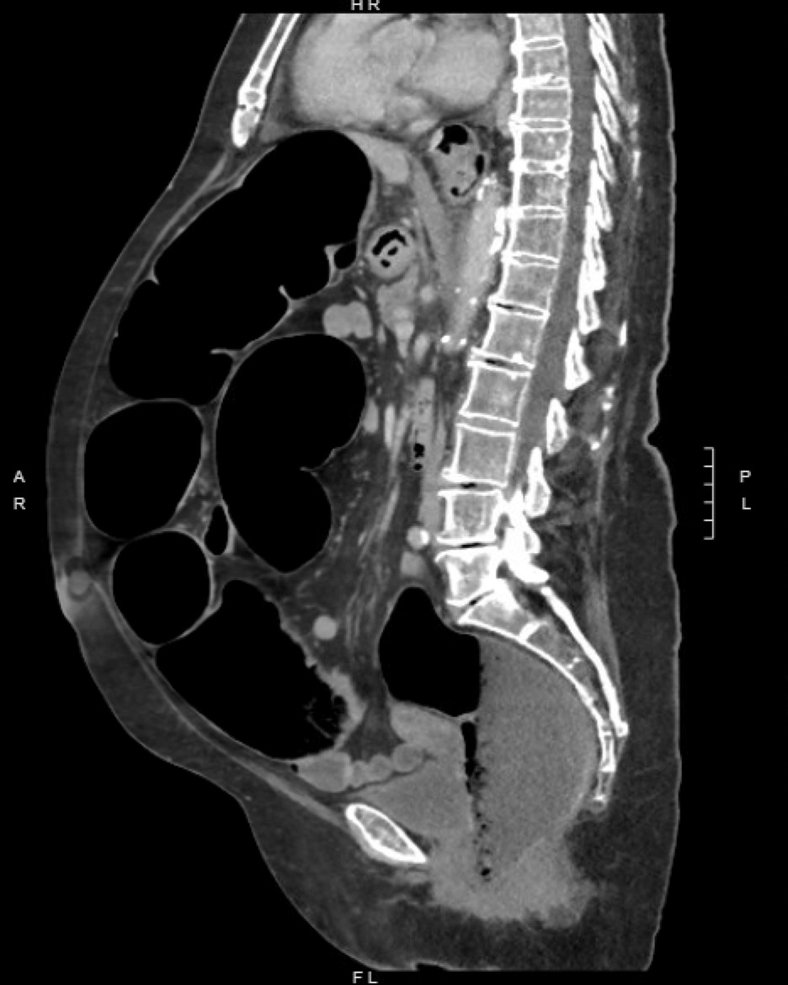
Sagittal view of computed tomography abdomen/pelvis revealing distention of large bowel.
